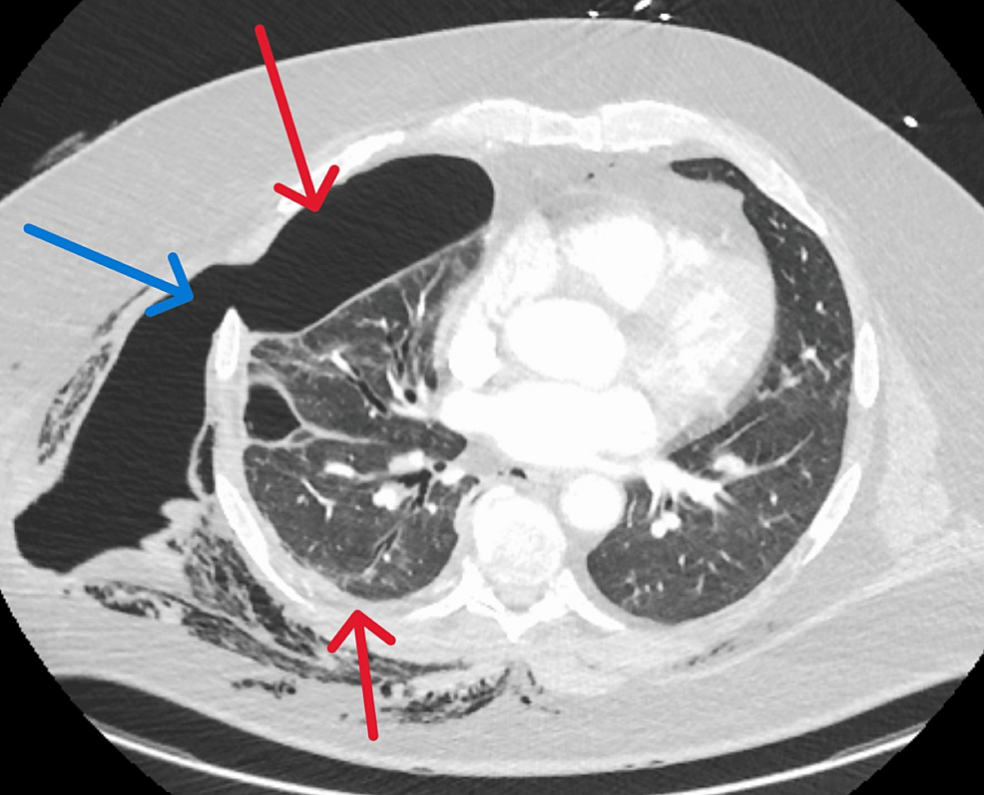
Computed tomography angiogram (CTA) chest The red arrows show the right hydropneumothorax. The blue arrow shows intercostal herniation of the pleura between the right fifth and sixth ribs out into the right lateral chest wall.
